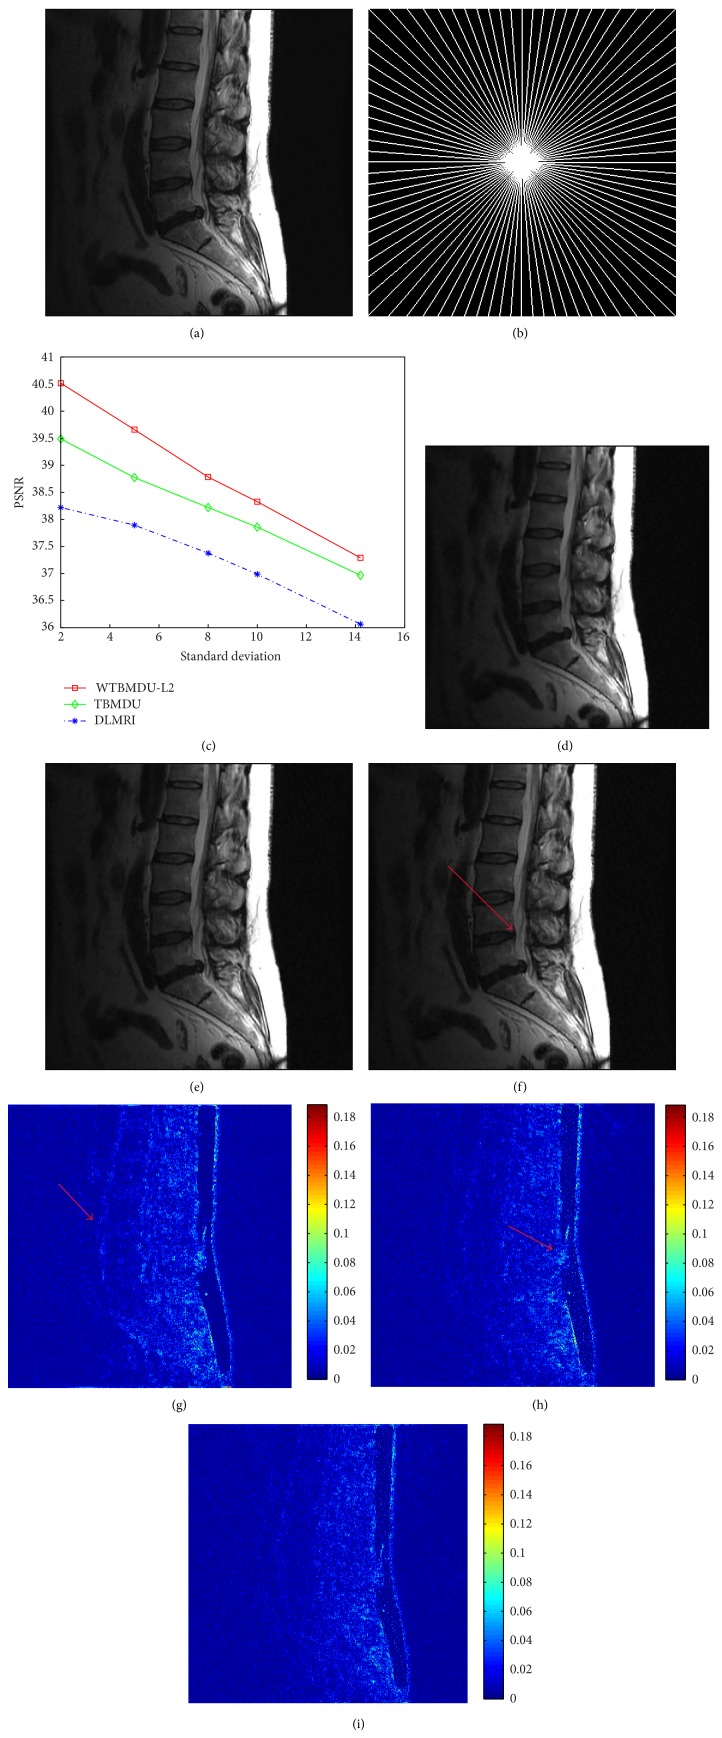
(a) The reference T2-weighted sagittal view of the lumbar spine. (b) Sampling mask in* k*-space with 6.09-fold undersampling. (c) PSNR versus noise level for DLMRI, TBMDU, and WTBMDU. ((d), (e), (f) and (g), (h), (i)) Reconstructions and corresponding error magnitudes for DLMRI, TBMDU, and WTBMDU with noise *σ* = 8.
